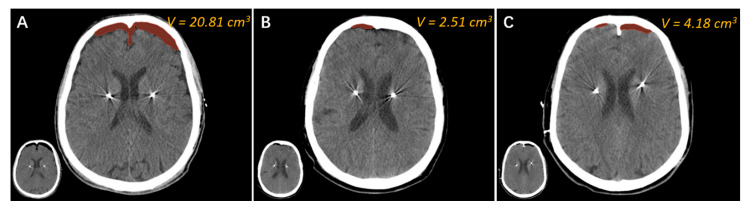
The comparison of three methods to reduce pneumocephalus and CSF loss. Representative intraoperative CT images of three patients who underwent bilateral electrode implantation, each with one of three burr hole-sealing approaches, are presented. (A) A 58-year-old male patient with Parkinson’s disease (PD) underwent bilateral STN-DBS electrode implantation in 2013. During the operation, bone wax was used to seal the burr hole. The air volume of the intraoperative CT images was 20.81 cm^3^. (B) A 65-year-old female patient with ET underwent bilateral Vim-DBS electrode implantation in 2021. The burr hole was filled with fibrin glue during the surgery. The air volume of the intraoperative CT images was 2.51 cm^3^. (C) A 60-year-old female patient with ET underwent bilateral Vim–PSA DBS electrode implantation in 2022. We adopted the “water sealing” method for this patient. The air volume of the intraoperative CT images was 4.18 cm^3^.
